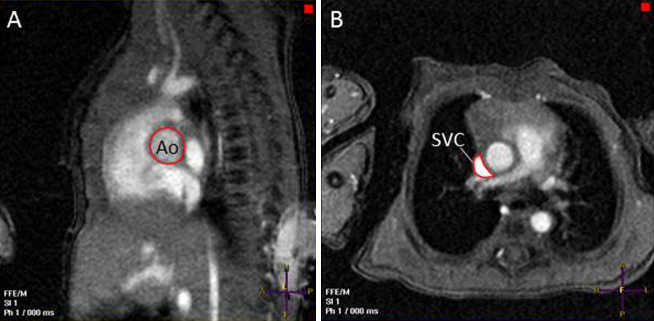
PC MRI quantification of (A) LVO at the level of the aortic valve and (B) superior vena caval flow at the level of the pulmonary trunk. *Ao*, Aorta; *SVC*, superior vena cava.
